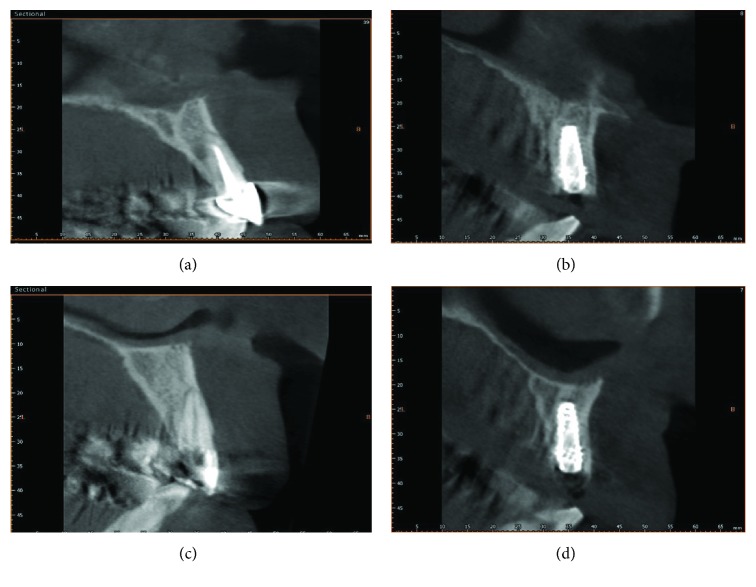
(a) Before extraction the CBCT image showing a thin buccal plate. (b) After 6 months of implant placement, the buccal plate was preserved and the gap was filled by new a bone. (c) Second clinical case. Before extraction, there is a thin buccal plate. (d) After 6 months of implant placement. Also in this case, the buccal plate was preserved.
